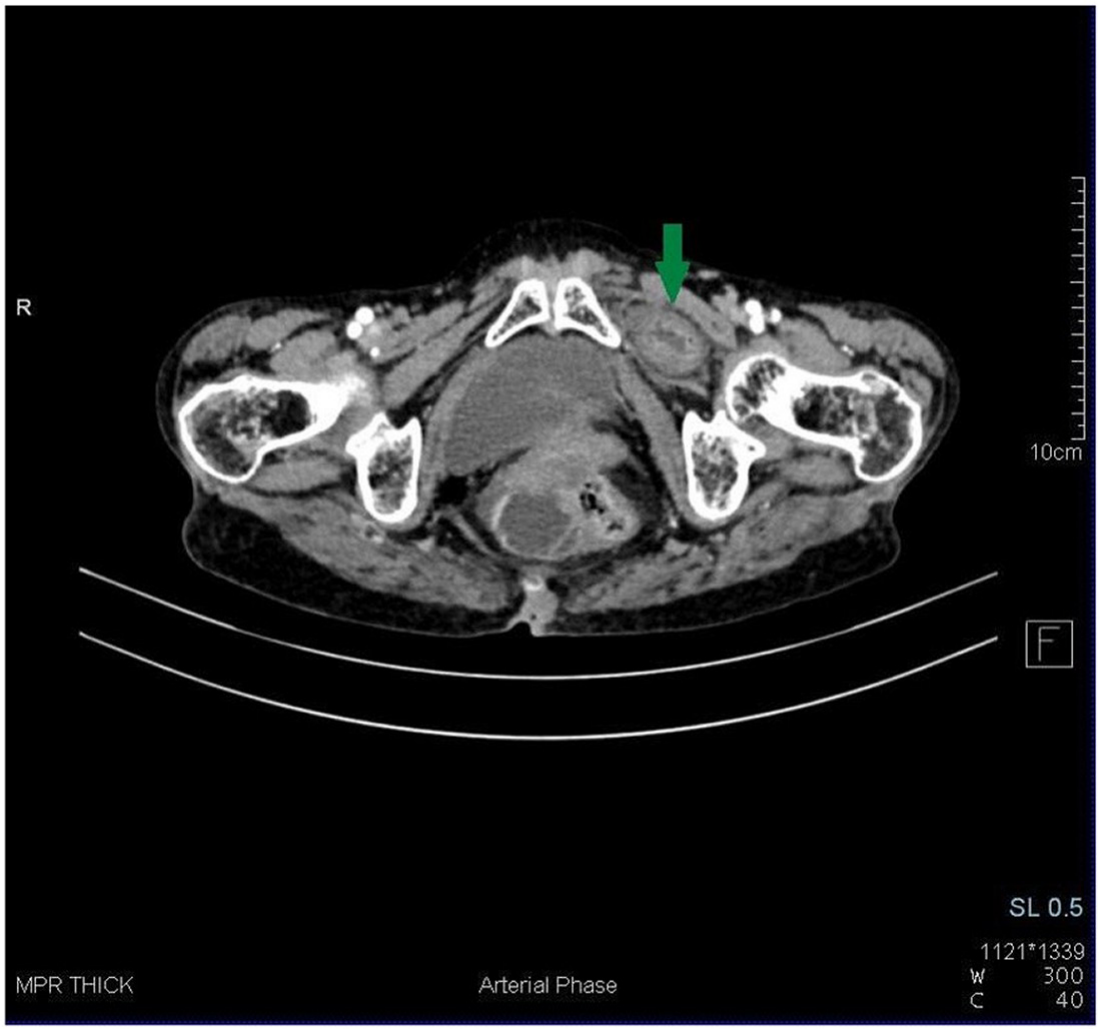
Arterial phase axial computed tomography showing a loop of small bowel (distal jejunum) herniating through the left obturator foramen between the left pectineus and obturator externus muscles. On the opposite side, three muscular layers are clearly visible around the obturator foramen: internal obturator, external obturator, and pectineus.
